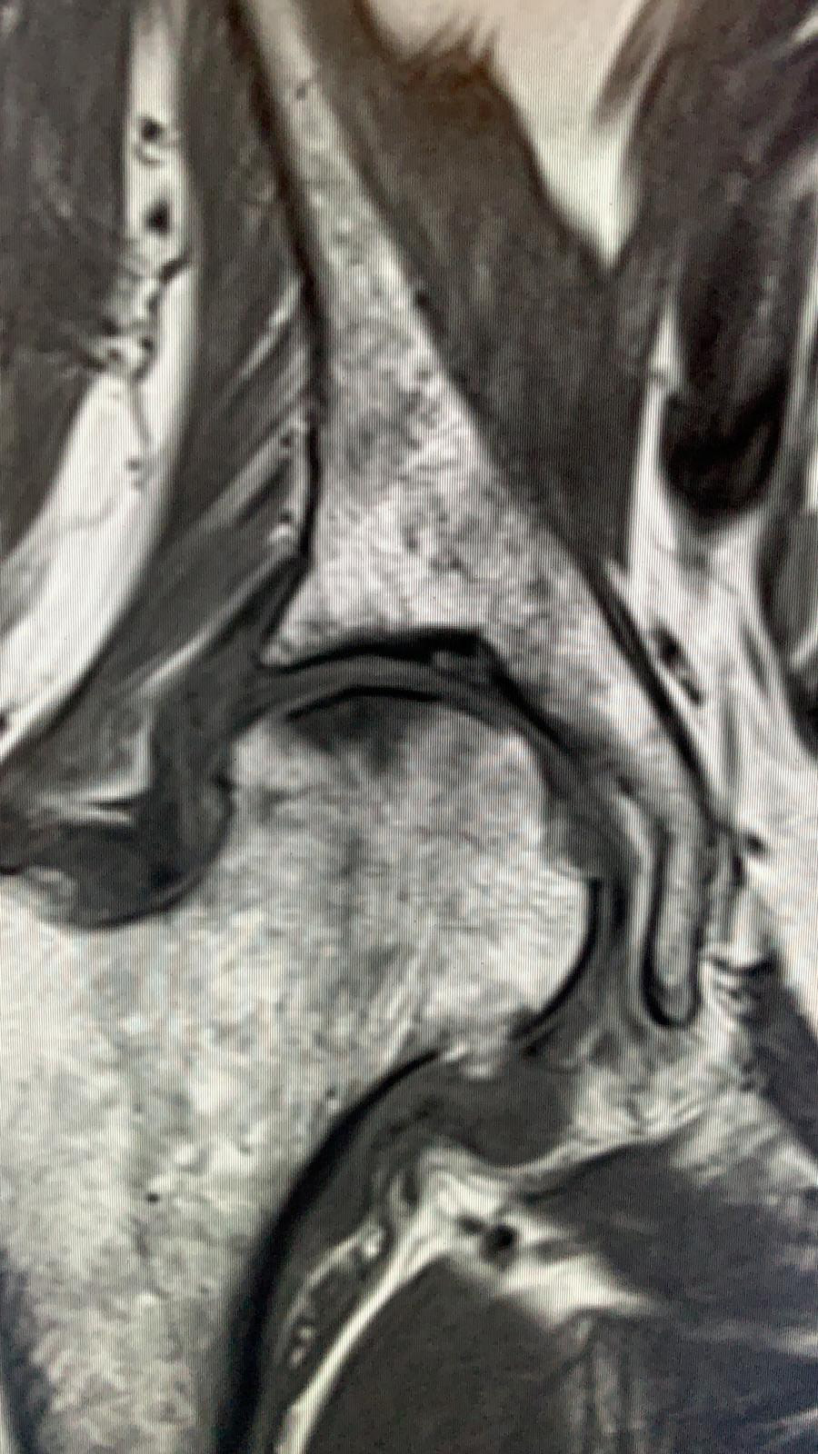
MRI Coronal T1 imaging of right hip demonstrating a linear low signal subchondral line in the superior femoral head consistent with subchondral insufficiency fracture.
